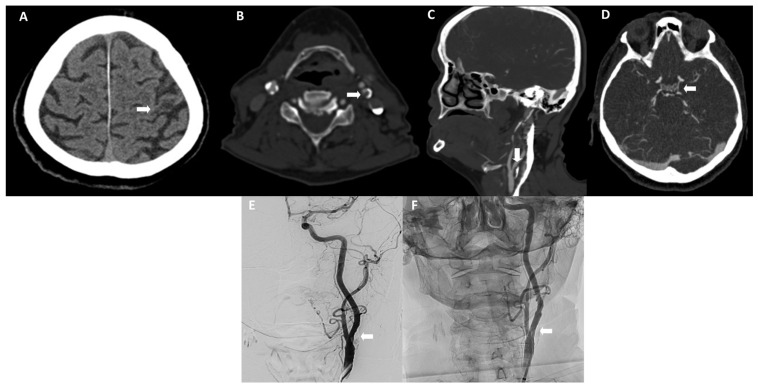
Imaging before neurological worsening. (A) A CT pre-procedure showing postischemic encephalomalacia in the left frontal region (arrow). (B,C) A CT angiography (CTA) pre-procedure showing a hemodynamically significant left internal carotid artery (ICA) stenosis of 80–90% (arrows). (D) A CTA pre-procedure showing a patent left posterior communicating artery. (E) A DSA showing a 50% left ICA stenosis (arrow). (F) The DSA showed a patent stent without residual stenosis at the end of the procedure (arrow).
